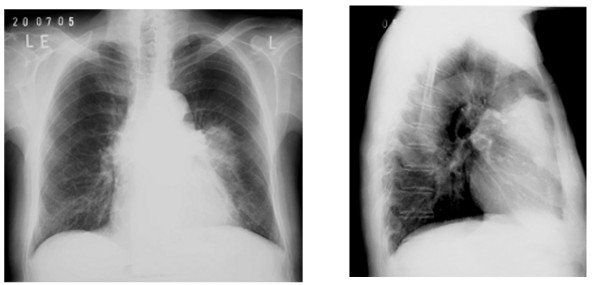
Chest x-ray in anterior-posterior projections showing an anterior-medial infiltrate of the left lung.
